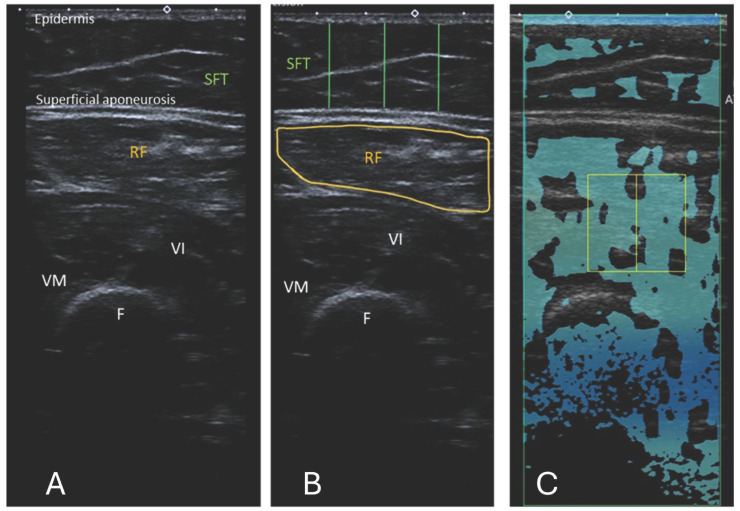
(A) Ultrasound B-mode image of the RF, musculus vastus intermedius, and medius. The distance between the epidermis and superficial aponeurosis is defined as subcutaneous fat tissue (SFT). (B) Segmented image of the RF, including the muscle cross-sectional area and the lines marking the thickness of the SFT from the lateral, central, and medial aspects of the RF. The measurement of the SFT was taken at 25%, 50%, and 75% of the total muscle visible on ultrasound, respectively. (C) Attenuation imaging of the RF. The green box indicates the area of interest (AOI), and the yellow box indicates the region of interest (ROI) placed within the quadriceps femoris muscles. RF = rectus femoris, VI = vastus intermedius, VM = vastus medialis, SFT = subcutaneous fat tissue, F = femur.
